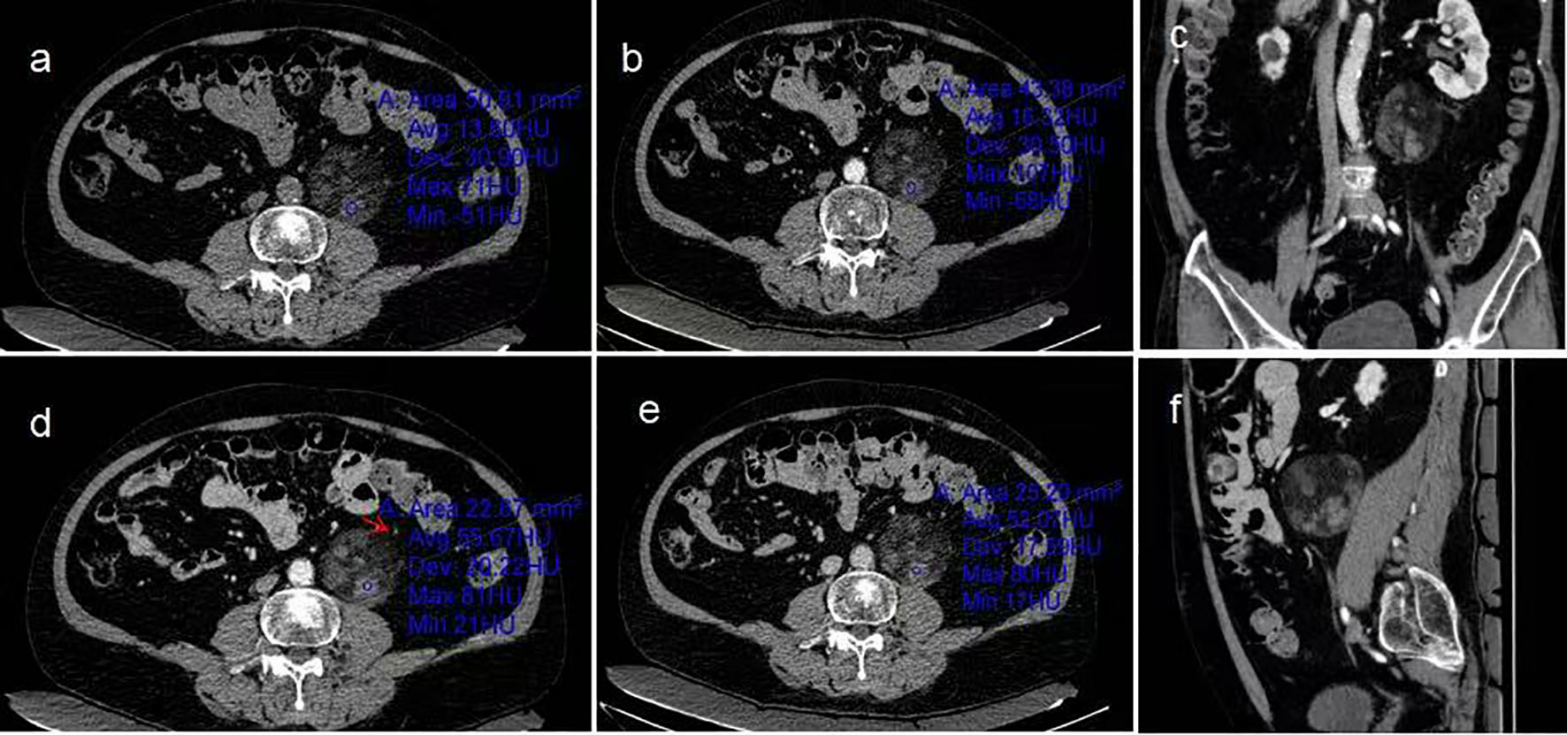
CT Non-contrast scan and enhancement. (a) Non-contrast abdominal CT scan shows a fat-containing heterogeneous density mass adjacent to the left side of the abdominal aorta in the retroperitoneum, (b–f) Contrast-enhanced abdominal CT scans demonstrate progressive moderate enhancement of some components within the mass, with no enhancement of the fatty components. The left ureter is compressed and displaced anterolaterally.
